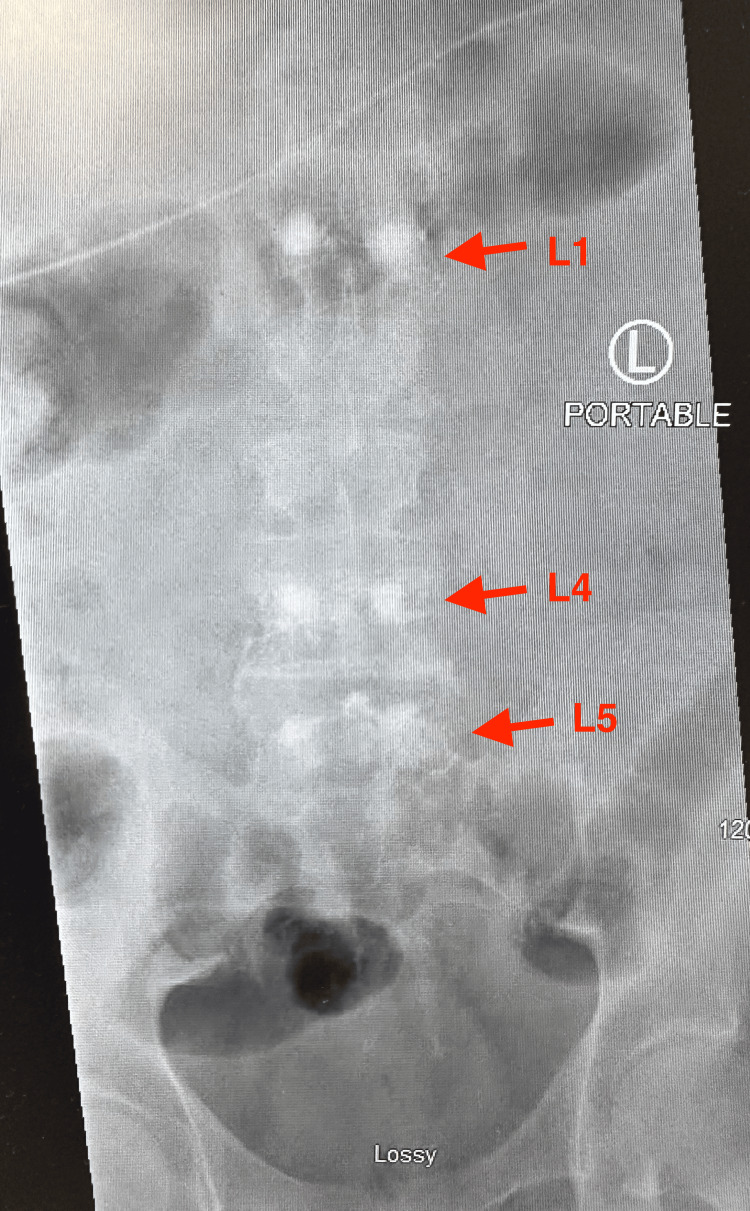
Portable anteroposterior lumbar spine X-ray demonstrating increased radiodensity consistent with compression deformities at L1, L4, and L5 (red arrows). Images captured using a smartphone camera due to limitations in accessing downloadable files. All identifiable information was removed.
